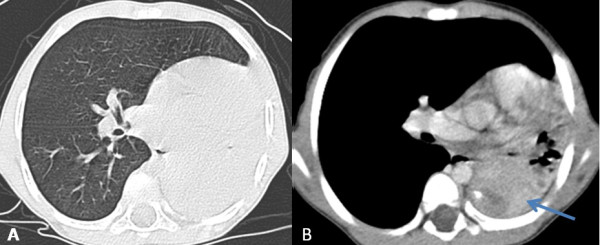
Chest Computed tomography axial cuts with parenchymal (A) and mediastinal (B) window showing a left lower lobe tumor containing a small calcification, associated with upper lobe atelectasis. The whole is responsible for the attraction of the mediastinum content to the left side.
